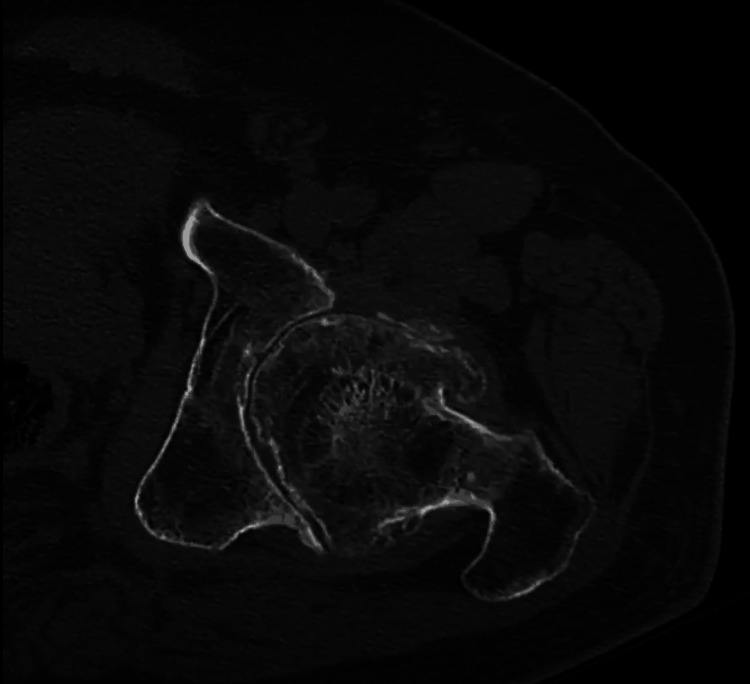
Axial CT scan of the left hip and femoral acetabular joint.
